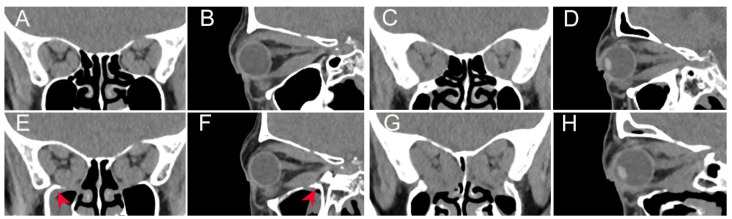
Orbital CT scans reveal that augmented orbital decompression surgery provides better decompression at the orbital apex. (A,B) Preoperative CT scans of a DON patient who underwent conventional orbital decompression surgery. (C,D) Preoperative CT scans of a DON patient who underwent augmented orbital decompression surgery. (E,F) Postoperative CT scans of the patient who underwent conventional orbital decompression surgery. (G,H) Postoperative CT scans of the patient who underwent augmented surgery. The arrows highlight the area of the orbital process of palatine bone (OPPB) that has been preserved following conventional orbital decompression surgery.
